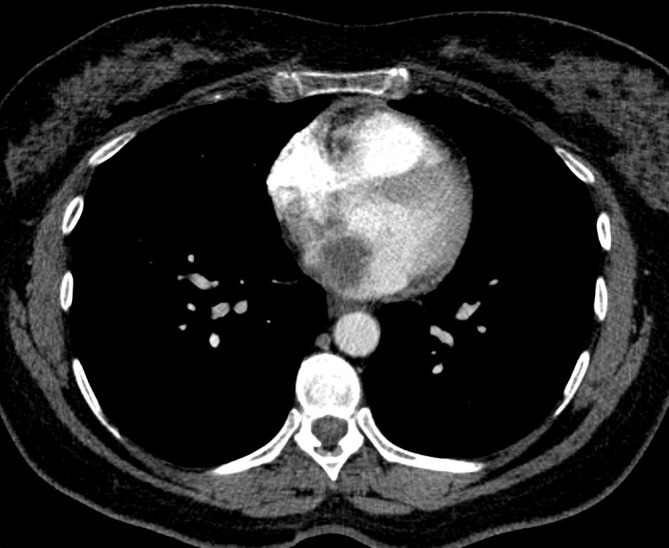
Post contrast, non-gated staging CT Chest showing a left atrial mass, concerning for cardiac myxoma.
